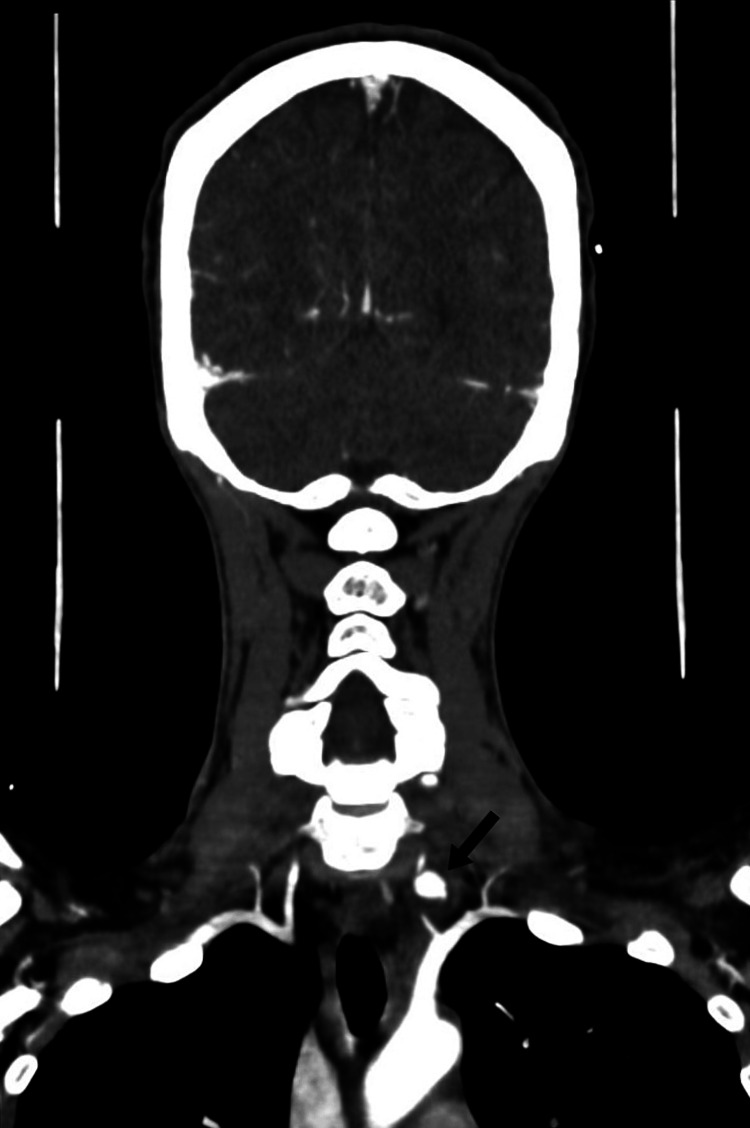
Preoperative computed tomographic angiography (coronal cut) demonstrating extravasation from the first segment of the left vertebral artery with pseudoaneurysm formation (arrow) CTA: computed tomography angiography
